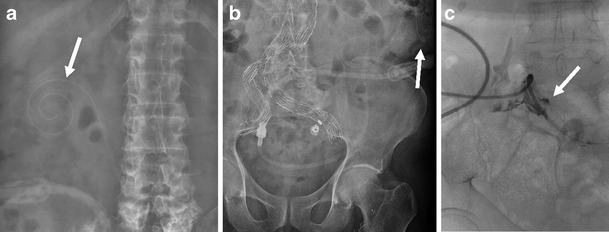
Abdominal radiographs demonstrating catheter tip migration superiorly from the pelvis to the right (a) and left (b) upper abdomen (*arrows*) in different patients and entrapment of the tip in another patient (c) with prevention of water soluble contrast from spilling freely into the peritoneal cavity on manual injection under fluoroscopy (*arrow*)
